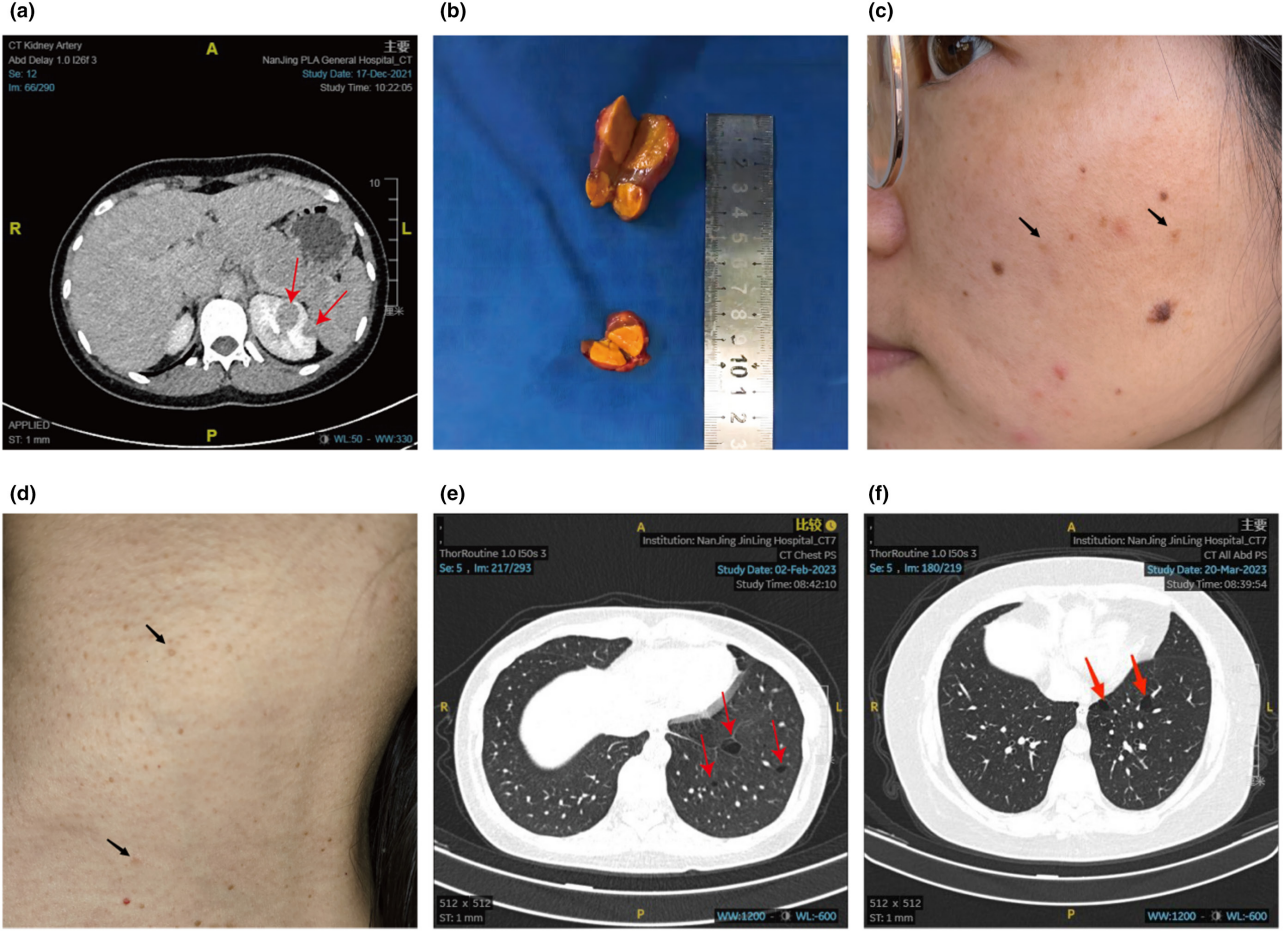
Clinical characteristics of the proband and her aunt. (a) Computed tomography of the proband's abdomen. The red arrow indicates multiple tumors in the left kidney. (b) Three renal tumors of the proband, ranging in diameter from 1.5 to 3 cm, after partial nephrectomy. (c) Multiple fibrous papules on the proband's face. (d) Multiple fibrous papules on the proband's neck. (e) Computed tomography of the proband's chest indicated multiple pulmonary bullae on the left side. (f) Chest computed tomography image of the proband's aunt showed multiple pulmonary bullae.
